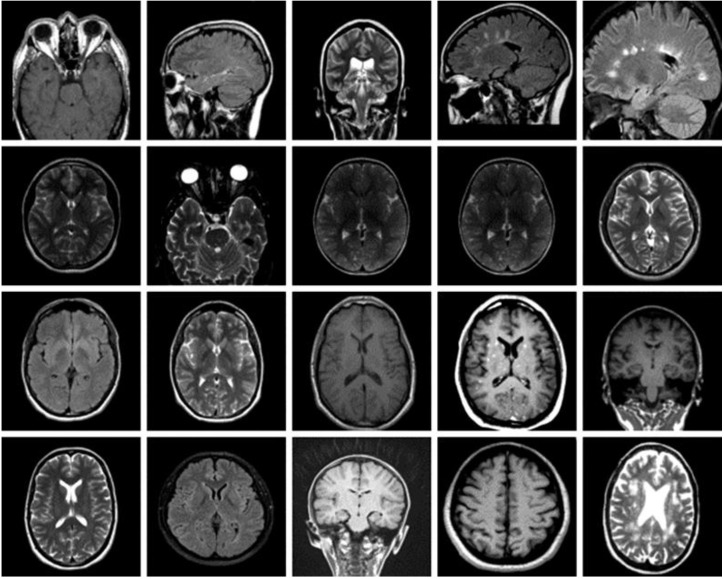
Examples of MRI scans with no tumor.
